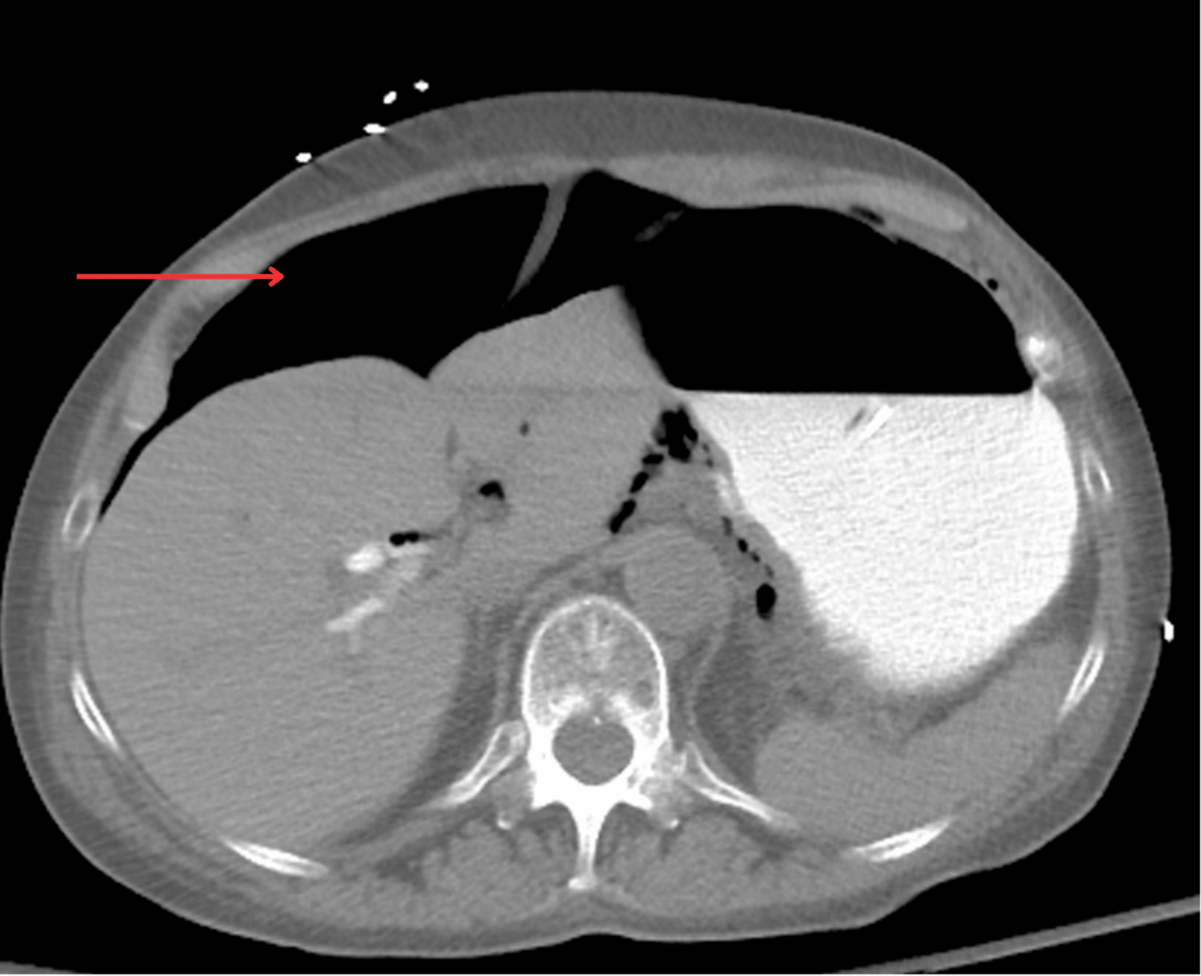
Abdominal CT showing pneumoperitoneum The red arrow indicates free air within the abdomen CT: computed tomography
